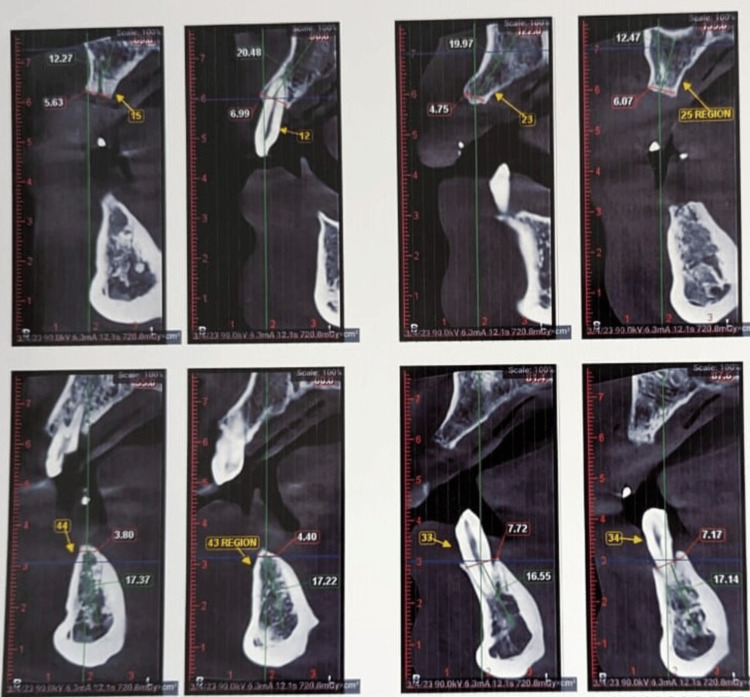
Cone-beam computed tomography in the region where implants were planned in 12, 15, 23, 25, 33, 34, 43, and 44 regions
